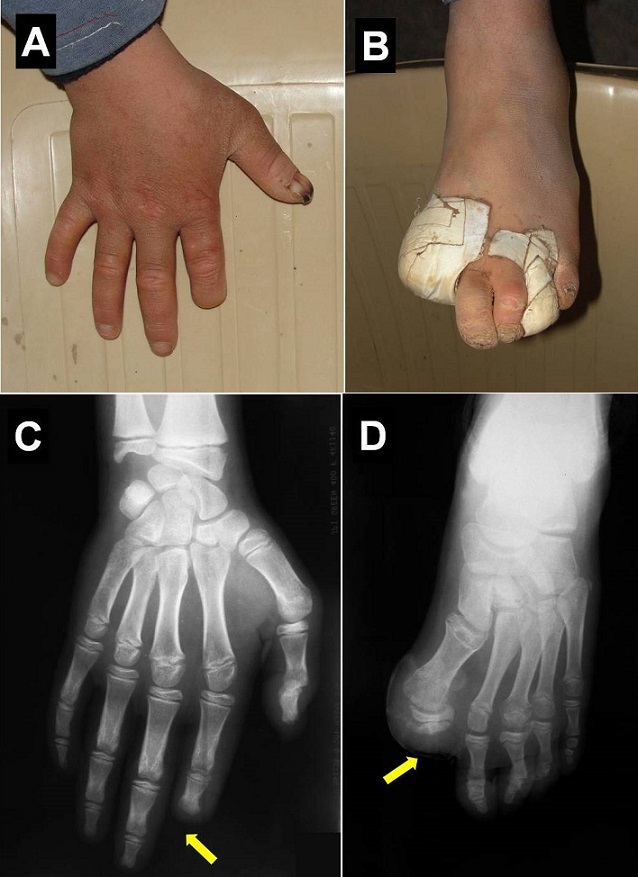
A) External photographs of right hand and left foot; B) showing self-mutilation of the distal phalanx of the second finger and the first toe was missing Hand (C) and foot (D) radiographs revealing destruction and amputation of the terminal part of the second right finger and the first left toe (arrows)
